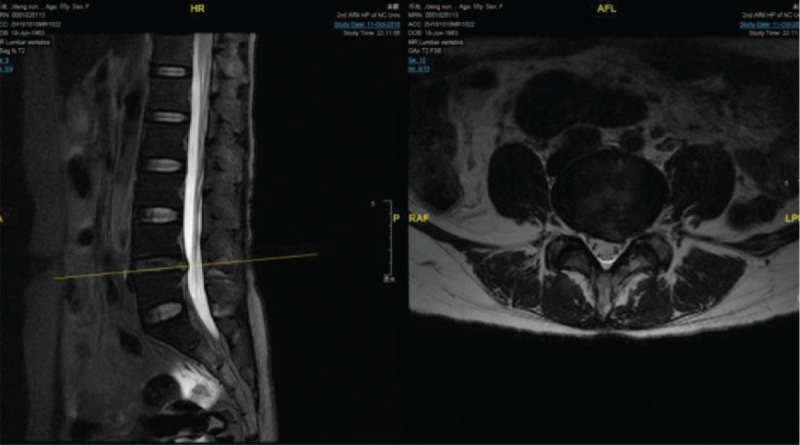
Preoperative magnetic resonance imaging (MRI) shows lumbar disc herniation at L4-L5.
